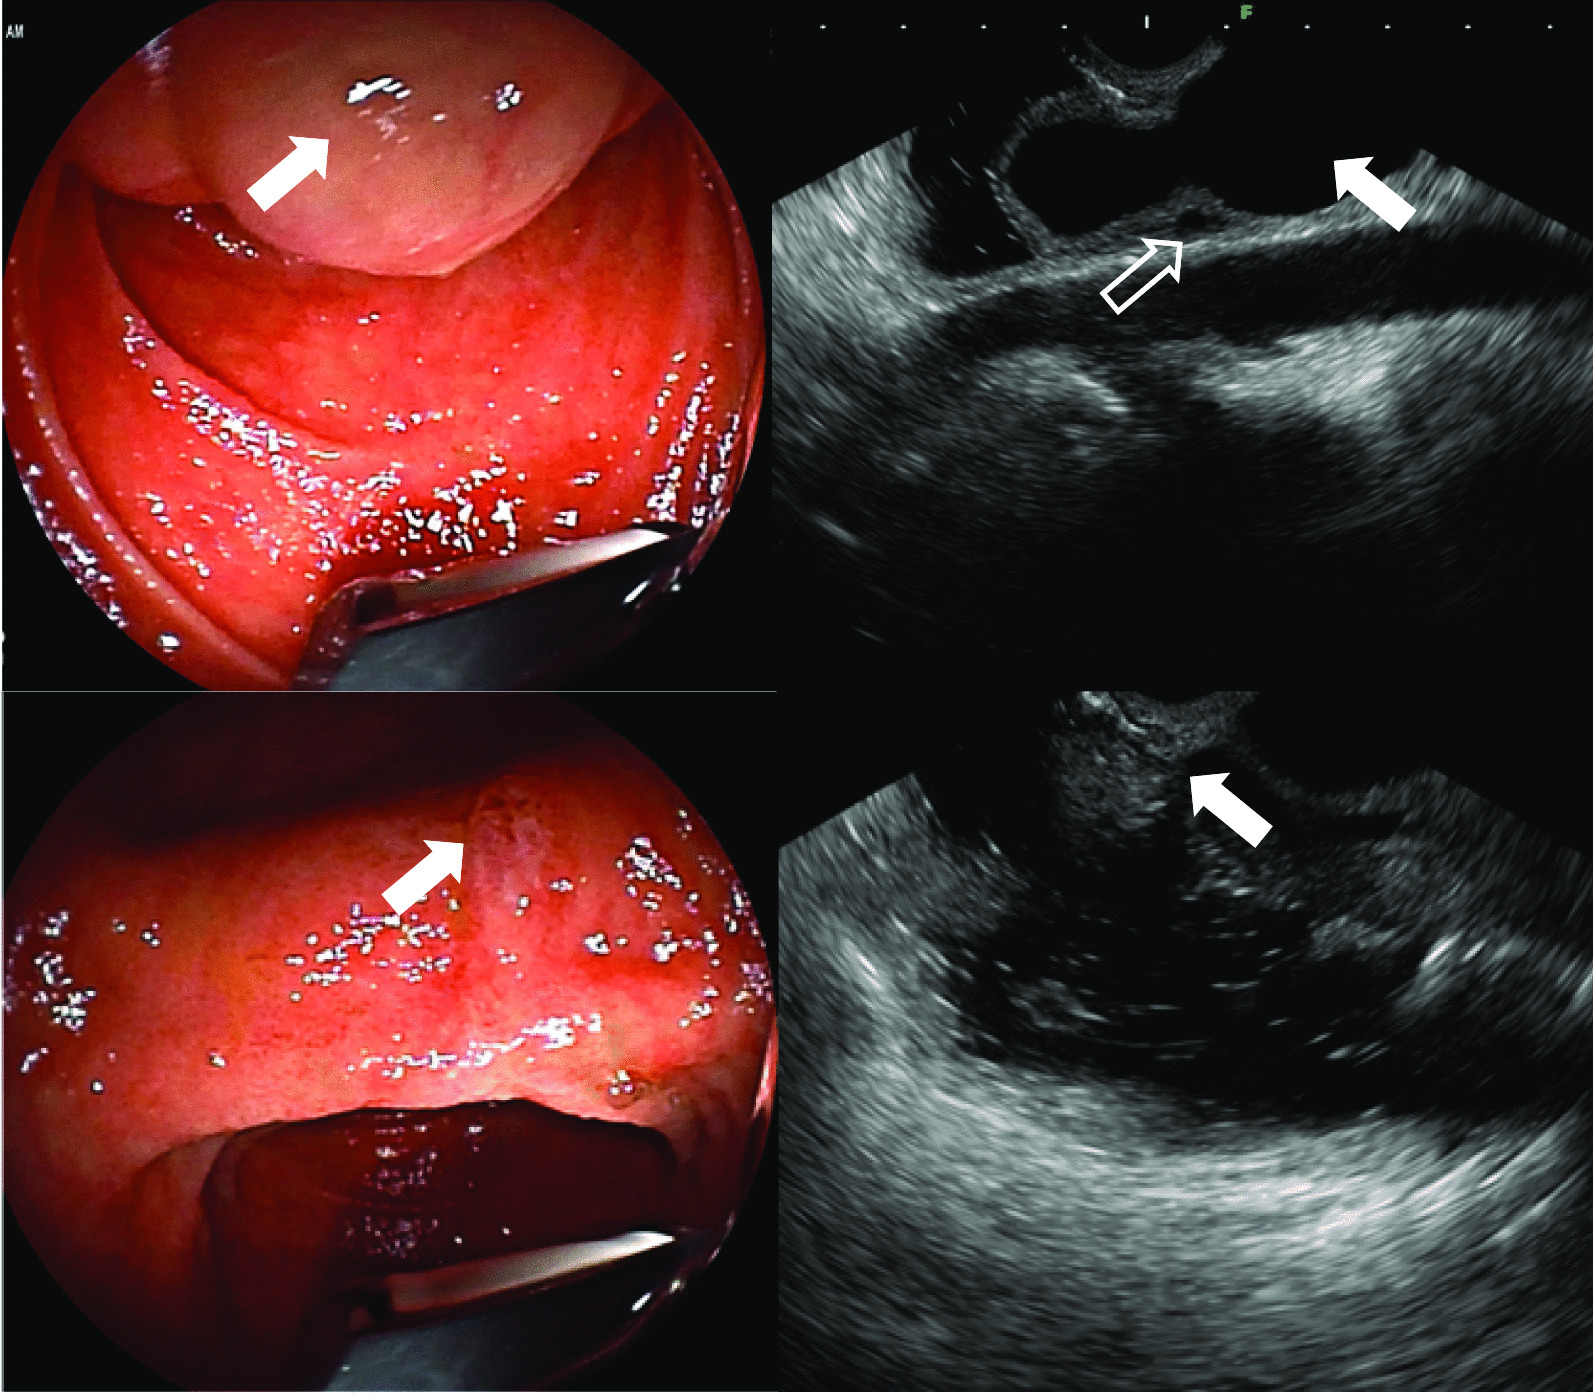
Endoscopic ultrasound shows that the end of the common bile duct expands and herniates into the duodenal cavity (②A arrow). The duodenal papilla located at the wall of the herniated intestinal cavity, which could be returned to its position after inflation (②B arrow). The common bile duct (②C arrow) compresses the pancreatic duct causing its secondary dilation (②C open arrow). No obvious abnormality of duodenal papilla (②D arrow)
